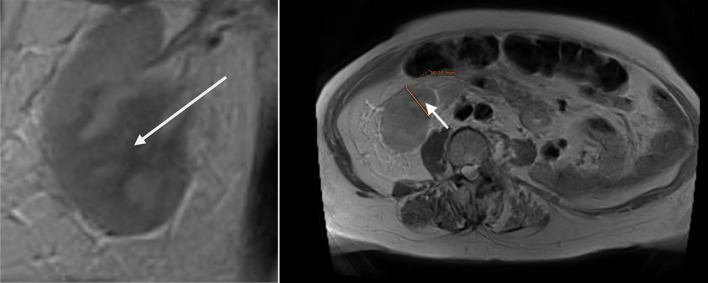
Upper abdomen magnetic resonance imaging (MRI) examination demonstrated tumor invading renal parenchyma (arrow).
